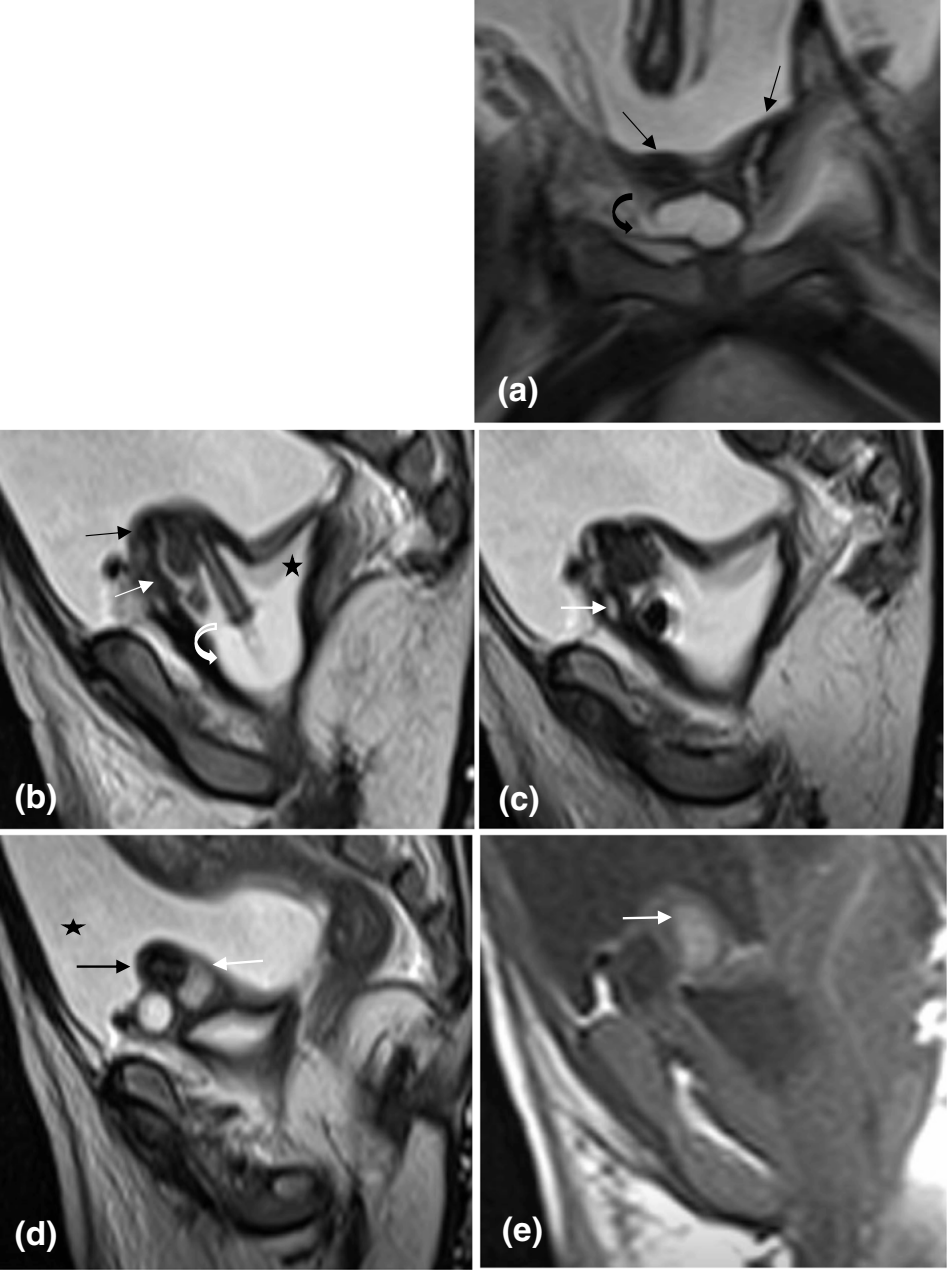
a Coronal T2 MRI of the pelvis showing two uterine bodies (black arrows) and cervices (not shown), in keeping with uterus didelphys. The dilated distal right ureter (curved arrow) lies anterior to the uterus (b) Left parasagittal T2 MRI of the pelvis showing the left uterine body (black arrow) and cervix (white arrow) opening into the vagina. Fluid (star) and a Foley catheter with a balloon (curved arrow) are seen in the vagina
